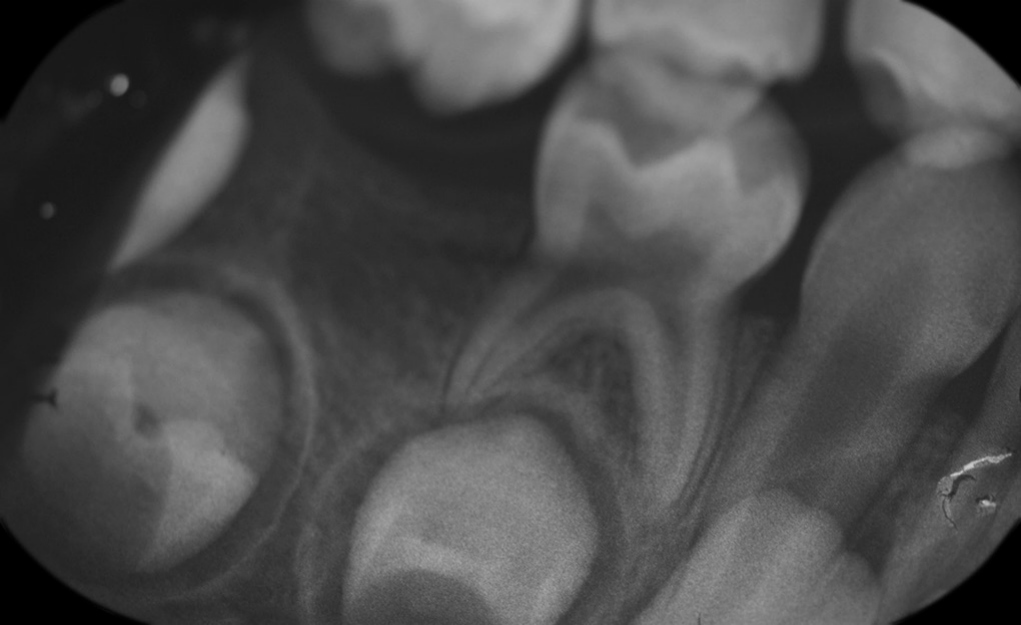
Periapical radiograph, 2 years later. Note: Normal development of the succedaneous tooth
